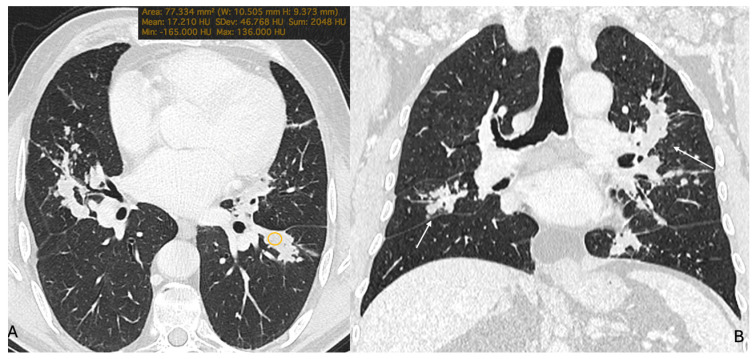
Axial lung-window CT (A) and coronal reformatted image (B) demonstrate a predominantly peribronchovascular nodular pattern, with clustered centrilobular/peribronchial nodules and branching opacities tracking along the bronchovascular bundles, most conspicuous in the middle lobe and lingula. Although this appearance can mimic infectious bronchiolitis or other inflammatory airway-centred processes, even for the the avarage positive density (Region of Interest rapresented by yellow circle in (A)), diagnosis is a biopsy-proven LP.
